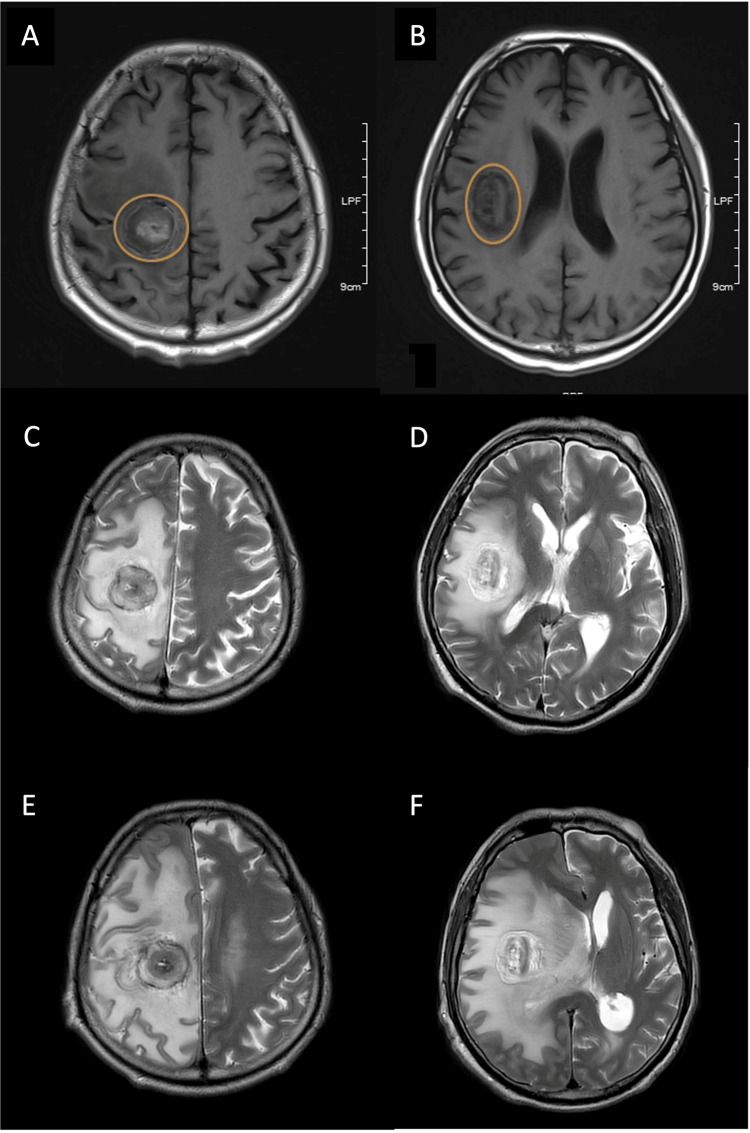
MRI scans at different time points (A, B) Axial T1 MRI of right frontal lobe glioblastomas (orange) three months post-operative; (C, D) axial T2 MRI tumor necrosis six months post-operative; (E, F) axial T2 MRI tumor shrinkage necrosis and vasogenic edema nine months post-operation
